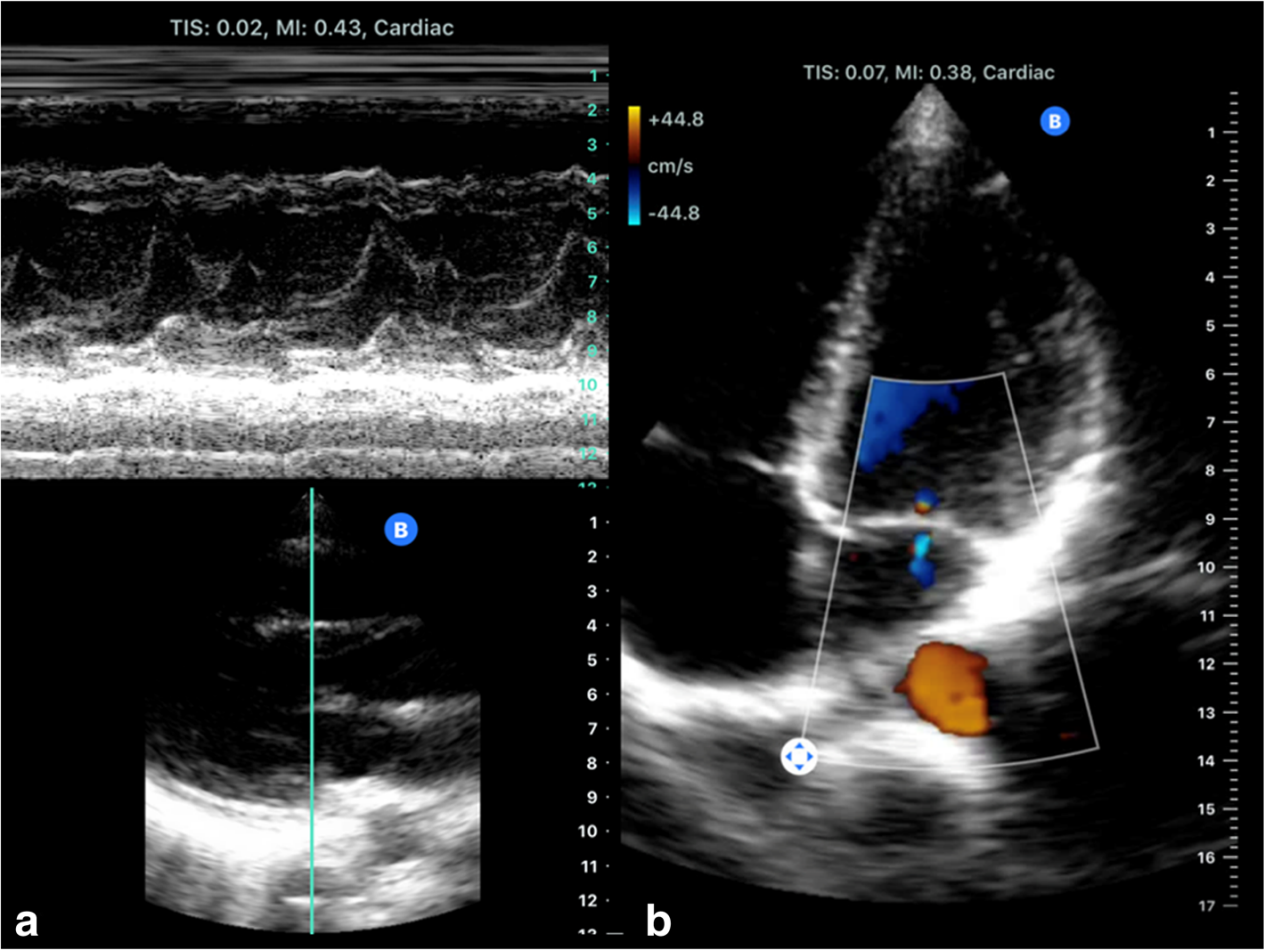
Butterfly’s iQ emulate phased transducers: Cardiac imaging including M-Mode (a) and four chamber view with colour Doppler (b)
